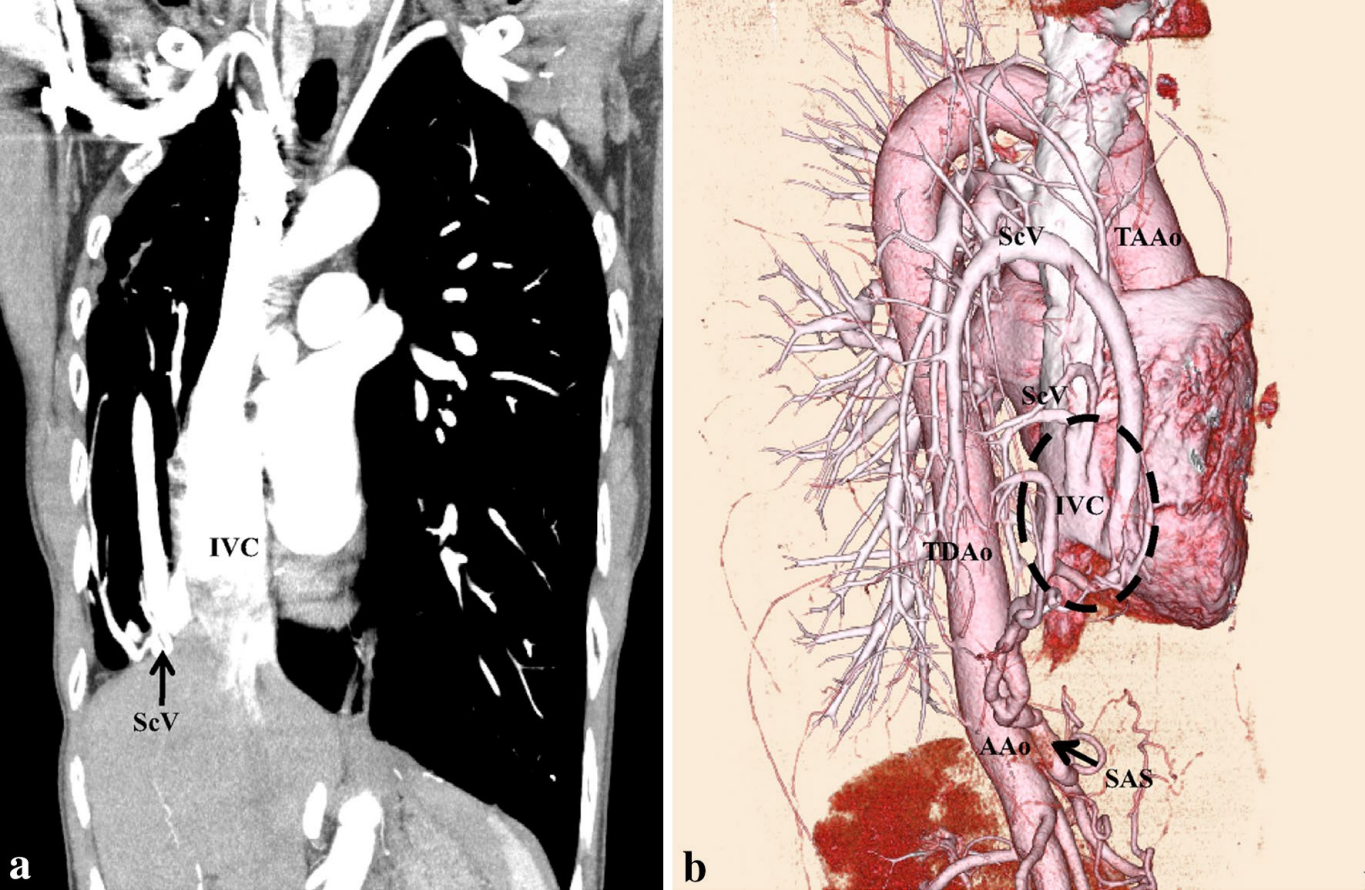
Coronal reconstruction of contrast-enhanced computed tomography showed scimitar vein drainage of the inferior vena cava (a). Three-dimensional volume-rendered computed tomography (*right anterior oblique view*) showed drainage of the inferior vena cava via several meandering scimitar veins (*dashed-line circle*) (b). *ScV* scimitar vein, *IVC* inferior vena cava, *TAAo* thoracic ascending aorta, *TDAo* thoracic descending aorta, *AAo* abdominal aorta, *SAS* systemic arterial supply
